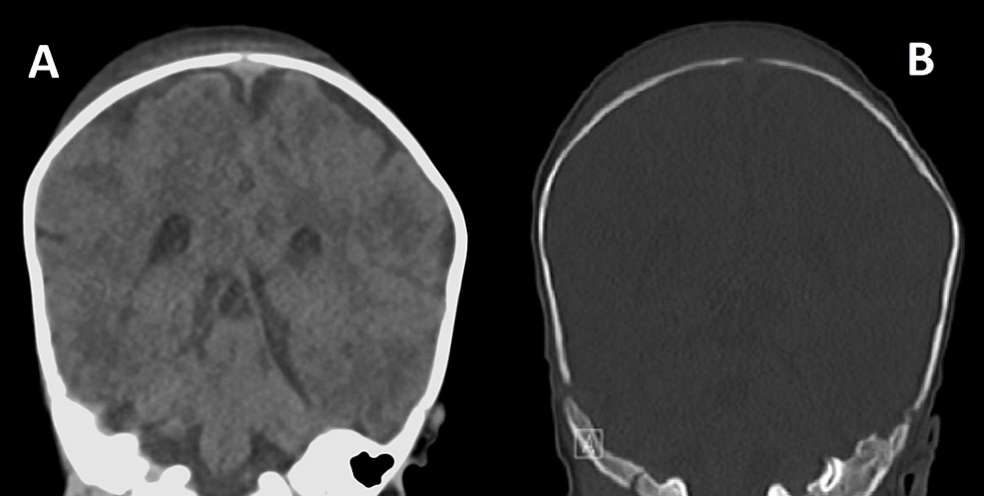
CT brain images of coronal orientation done on admission of the case. A (left image) soft tissue image showing a subaponeurotic scalp swelling crossing the sagittal suture line with no associated intracranial pathology underneath. B (right image) Bone window of the same image showing no detectable skull fractures related to the scalp swelling.
